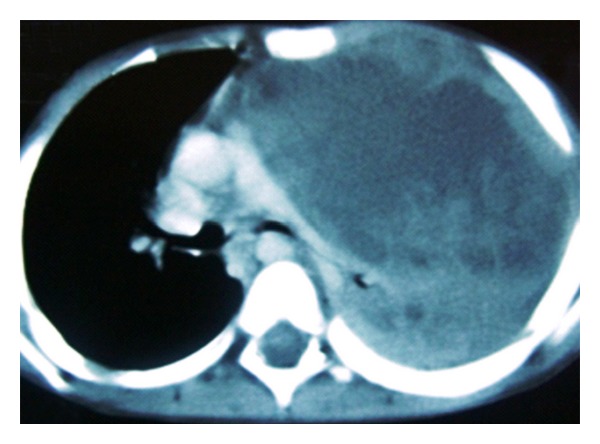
CT chest revealing a huge mass occupying the entire left hemithorax with mediastinal shift to opposite side (fat is not delineated nicely).
